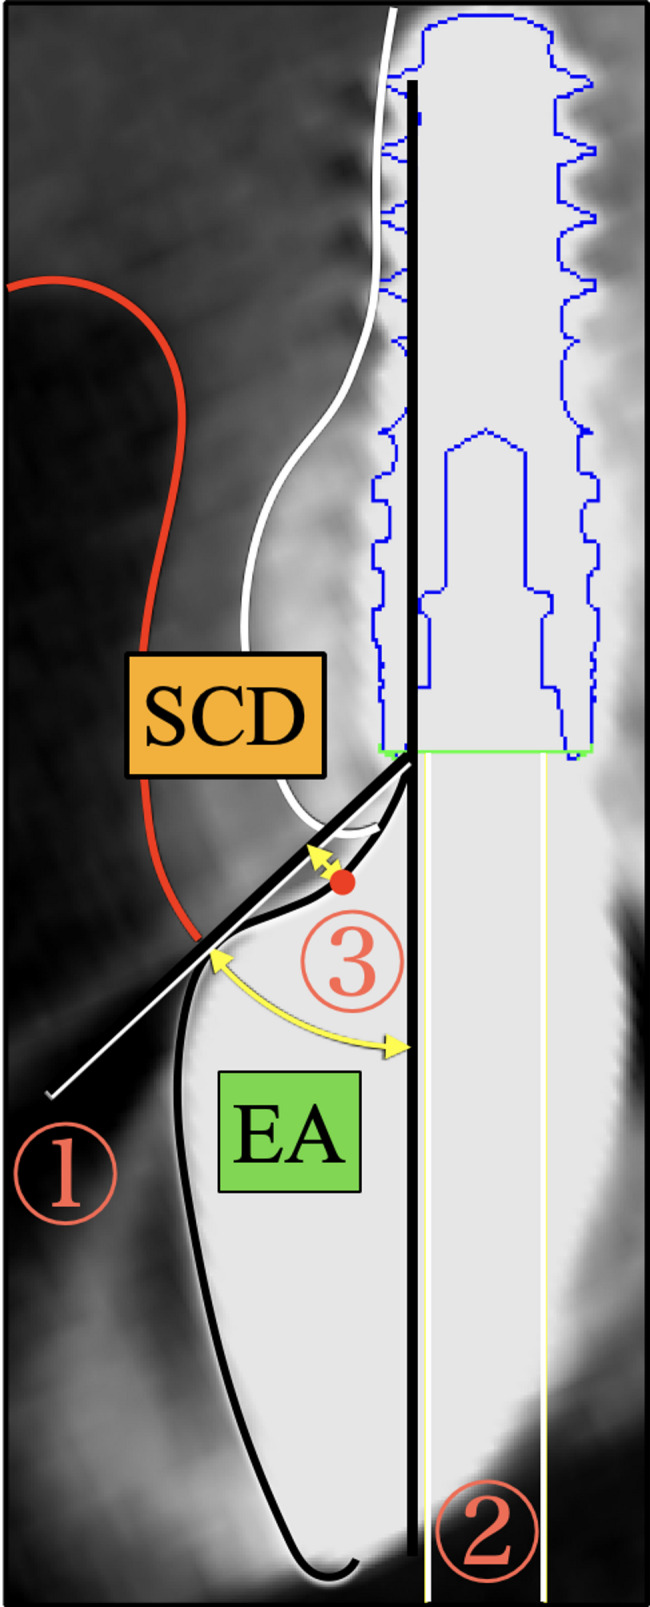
Measurement of labial transmucosal morphology (subgingival contour) on CBCT images. Emergence angle (EA): angle between tangent drawn from implant-abutment junction to superstructure ① and implant axis ②. Subgingival contour distance (SCD): distance from deepest point of subgingival contour of superstructure ③ to perpendicular line from tangent at implant-abutment junction ①
